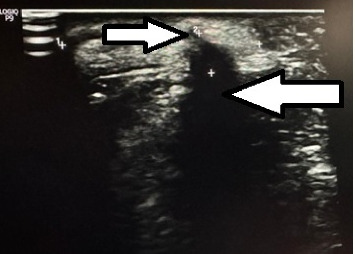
Ultrasound of the facial swelling showing a finger-like projection arising from the border of the mandible with a hyperechoic structure internally at the base (upper arrow) casting a posterior acoustic shadow (lower arrow).
